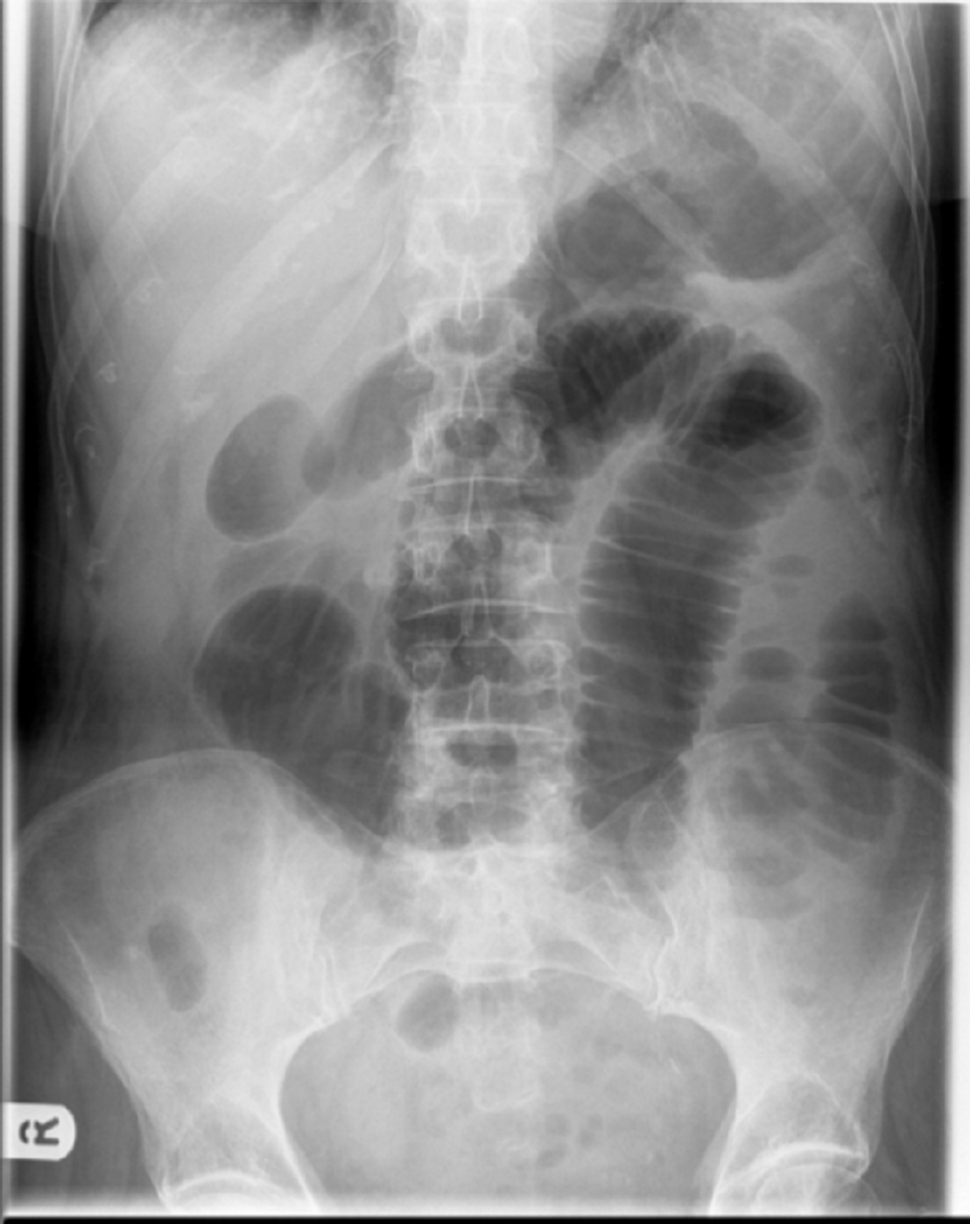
Plain abdominal radiograph showing signs of small bowel obstruction with dilated small bowel loops
